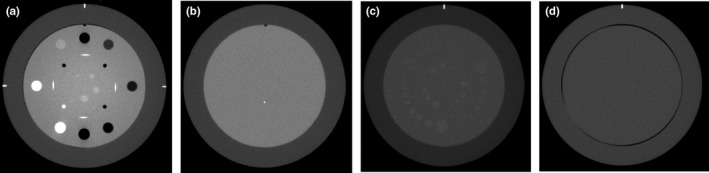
CT images from left to right of (a) the sensitometry module (CTP404), (b) the high‐resolution module (CTP528), (c) the low‐contrast module (CTP515), and (d) the uniformity module (CTP486).
